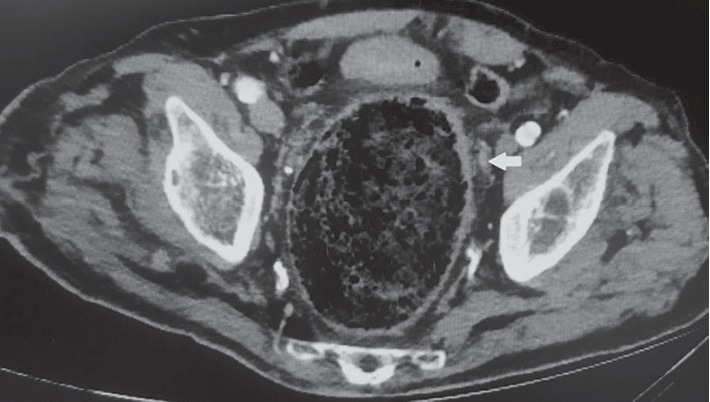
Computerized tomographic (CT) scan of the abdomen and pelvis. The left ureter (arrow) distal to the level of obstruction was not dilated.
